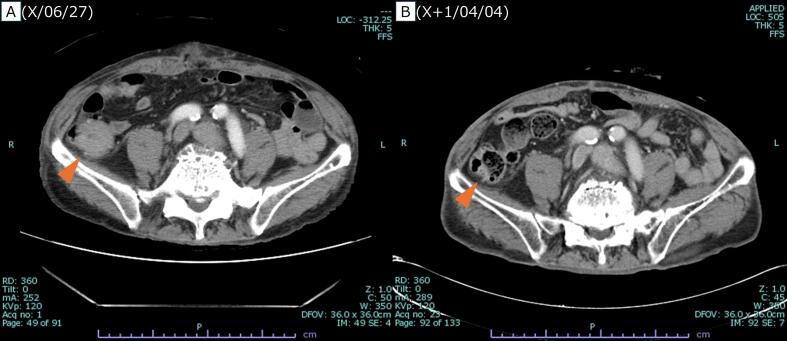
Preoperative CT for ileocecal resection. Contrast-enhanced CT showed shrinkage of the cecal cancer after systemic chemotherapy. A, Pre-chemotherapy. B, Post-chemotherapy.
